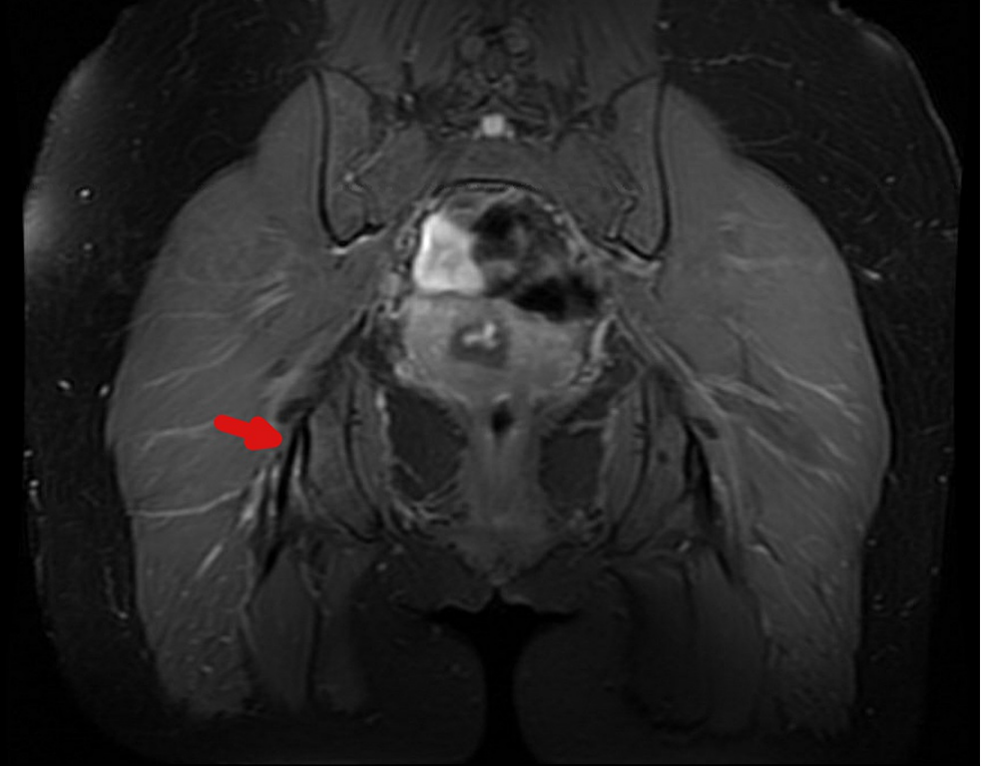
Coronal proton density (PD) fat-saturated (FS) MRI of bilateral hips at the level of the ischial tuberosities and lateral hamstring origins On the right, a high-intensity signal at the origin is consistent with high-grade partial tearing and tendinopathy (arrow) MRI: magnetic resonance imaging
